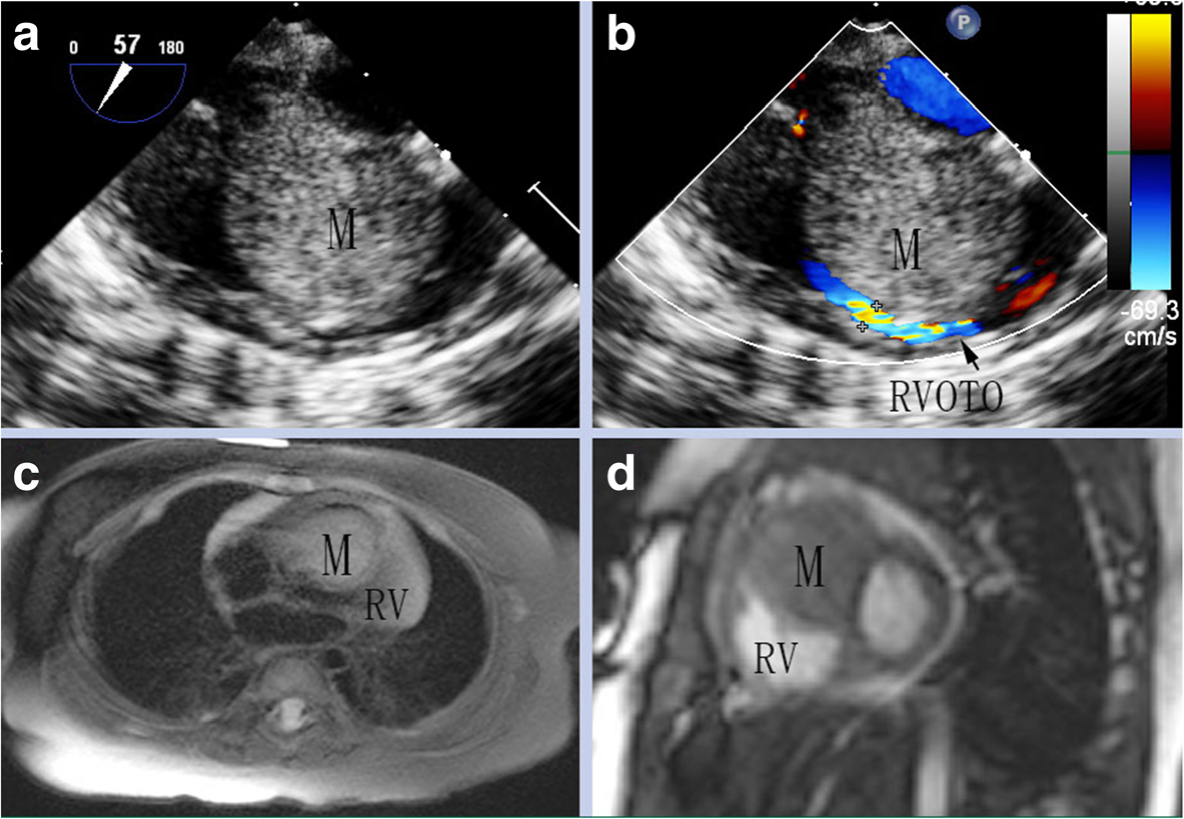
Image information demonstration the mass in the RV. a Transesophageal echocardiography. b Right ventricular outflow tract obstruction. c, d The Cardiac magnetic resonance imaging. M indicates mass, RV indicates right ventricle, RVOTO indicates right ventricular outflow tract obstruction
